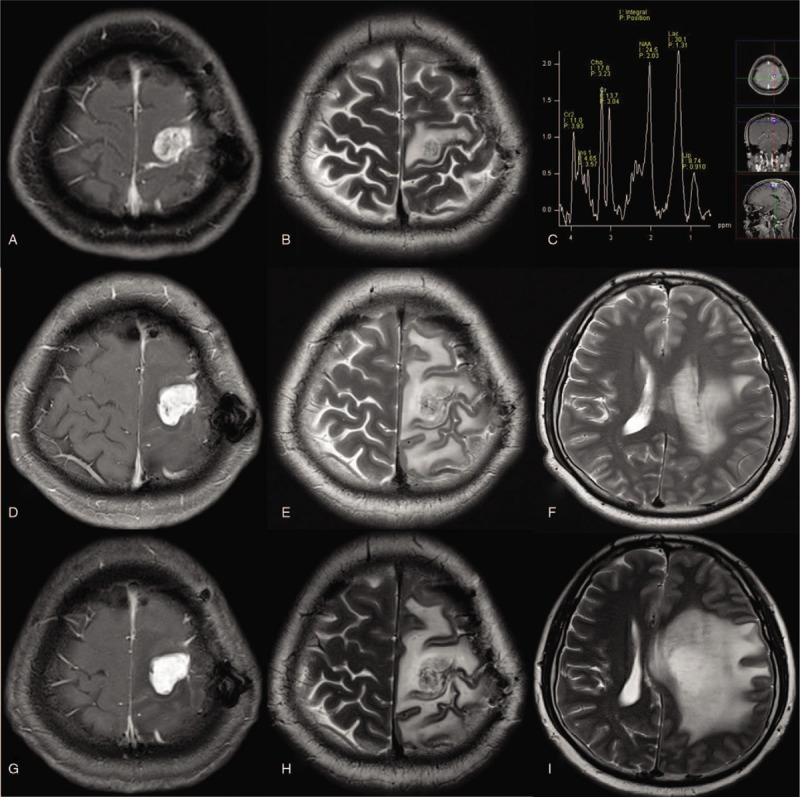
MRI at 3 months after GKRS demonstrated increased size of the tumor and peritumoral edema with lactate peak suggesting radiation necrosis. (A–C) Serial follow-up MRIs obtained at 9 months (D–F) and 18 months (G–I) after GKRS shoed progressively worsened peritumoral edema with midline shifting regardless of steroid treatment.
